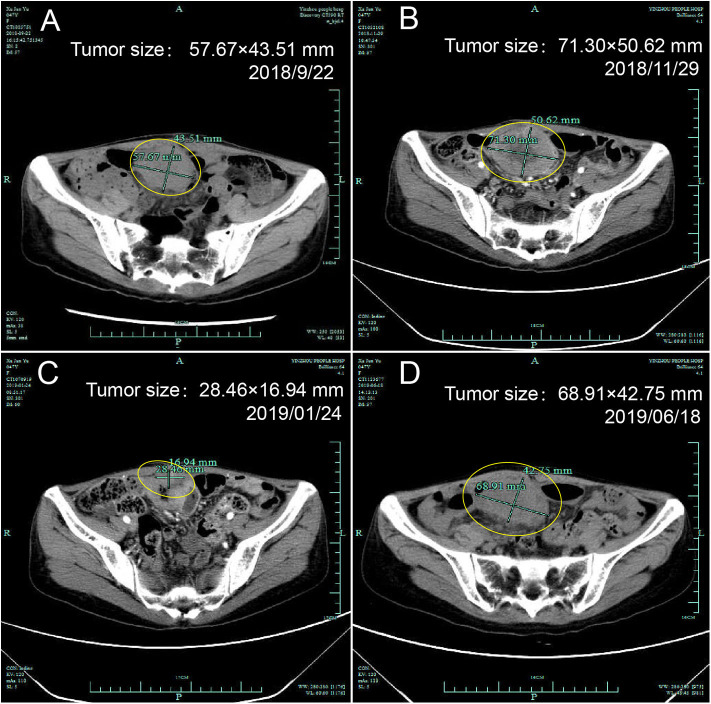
Computed tomography of the ovary before and after initiation of nivolumab treatment. (A) Tumor status before treatment, (B) Pseudo-progression within the first 2 months of treatment, (C) Partial response after treatment, (D) Progression.
